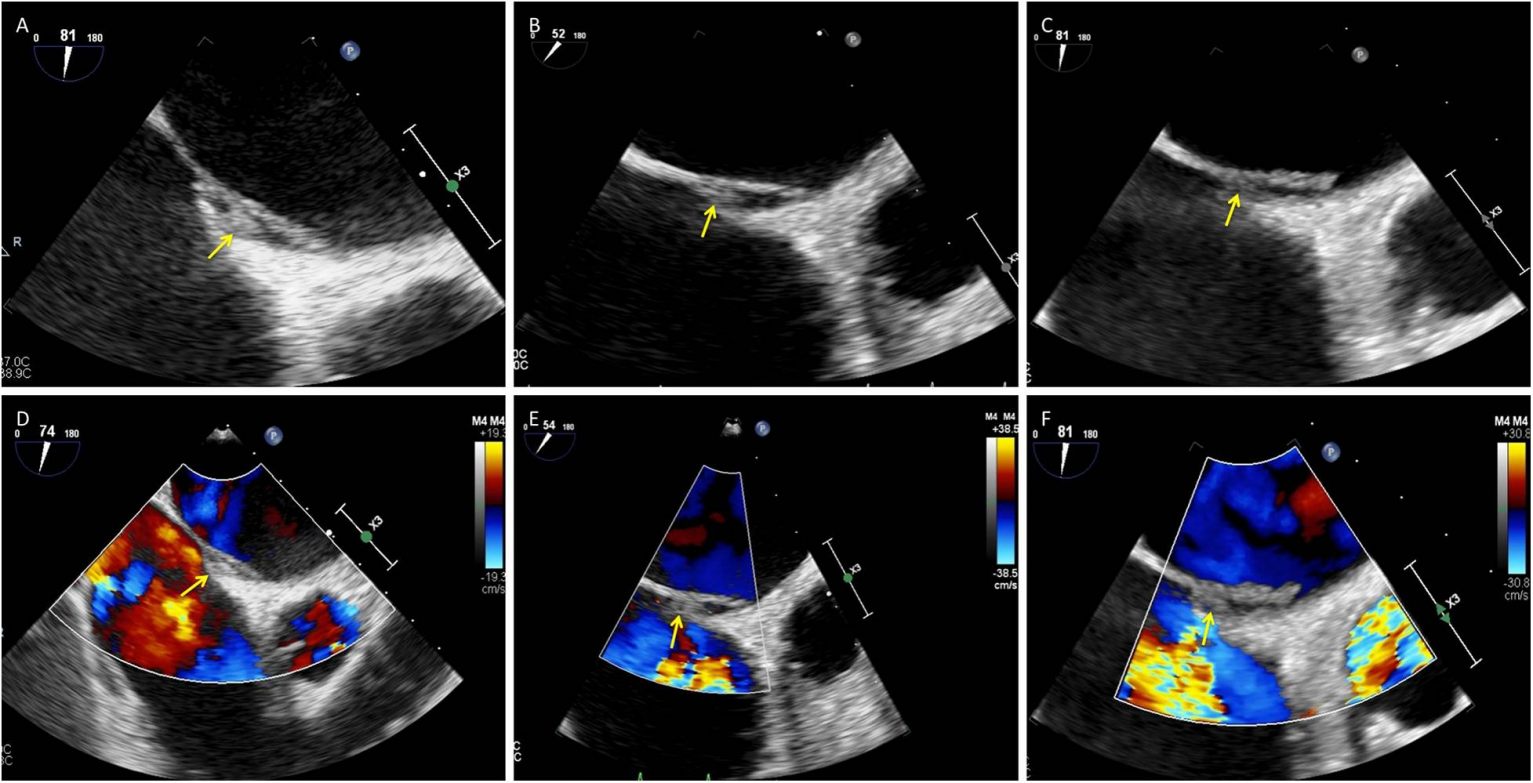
Small presumed *in situ* thrombus within the PFO tunnel with interval regression under oral anticoagulation: serial TEE. (A–C) Grayscale TEE images (mid-esophageal views) obtained at baseline, day 54, and day 141 show a small, relatively fixed thrombus within the PFO tunnel (arrows). The thrombus measured 3.1 × 9.1 mm at baseline (A), 2.3 × 5.6 mm on day 54 (B), and 2.1 × 4.6 mm on day 141 (C). (D–F) Corresponding color Doppler images at the same time points depict interatrial flow across the PFO (arrows). PFO, patent foramen ovale; TEE, transesophageal echocardiography; RA, right atrium; LA, left atrium.
